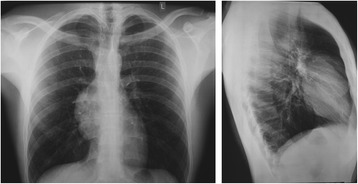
Chest X-ray shows a homogenous opacity in the *right mid upper zone* presenting no calcification
